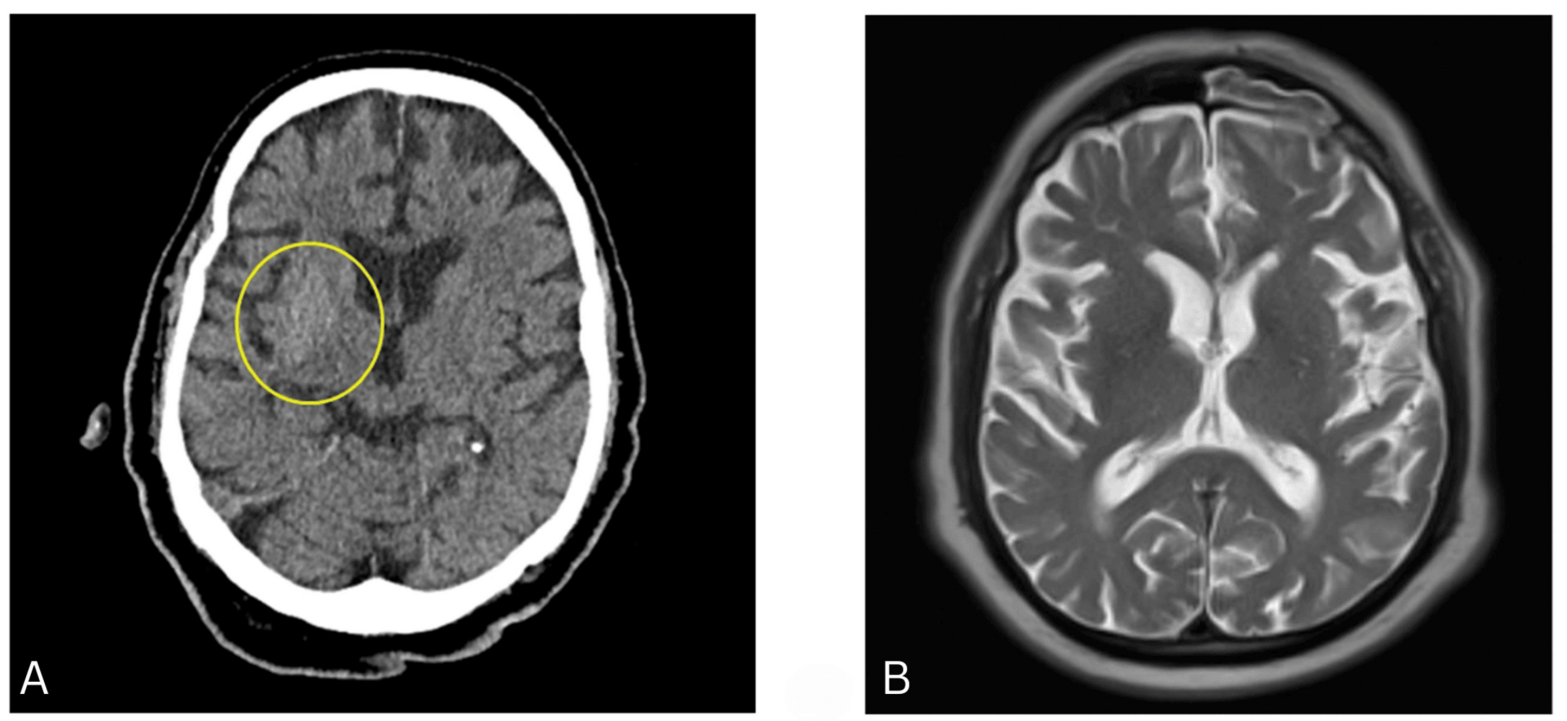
Non-contrast CT head scan (A) and T2 weighted MRI head scan (B) conducted one week apart (axial view) CT: computed tomography, MRI: magnetic resonance imaging
